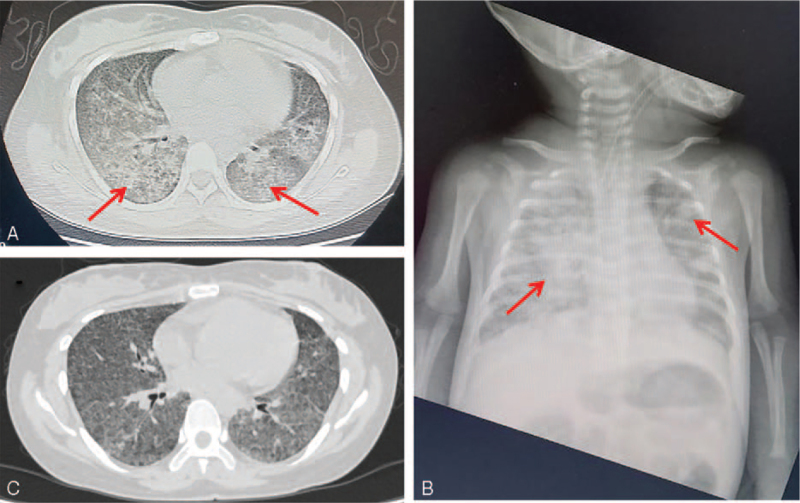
(A) Chest computed tomography (CT) of the patient (mother) revealing diffuse interstitial changes and both lungs’ exudation (red arrow). (B) The chest x-ray of the patient's infant daughter showing multiple patchy shadows in both lungs (red arrow). (C) Chest CT of the patient (mother) after 9 months of anti-tuberculous therapy.
